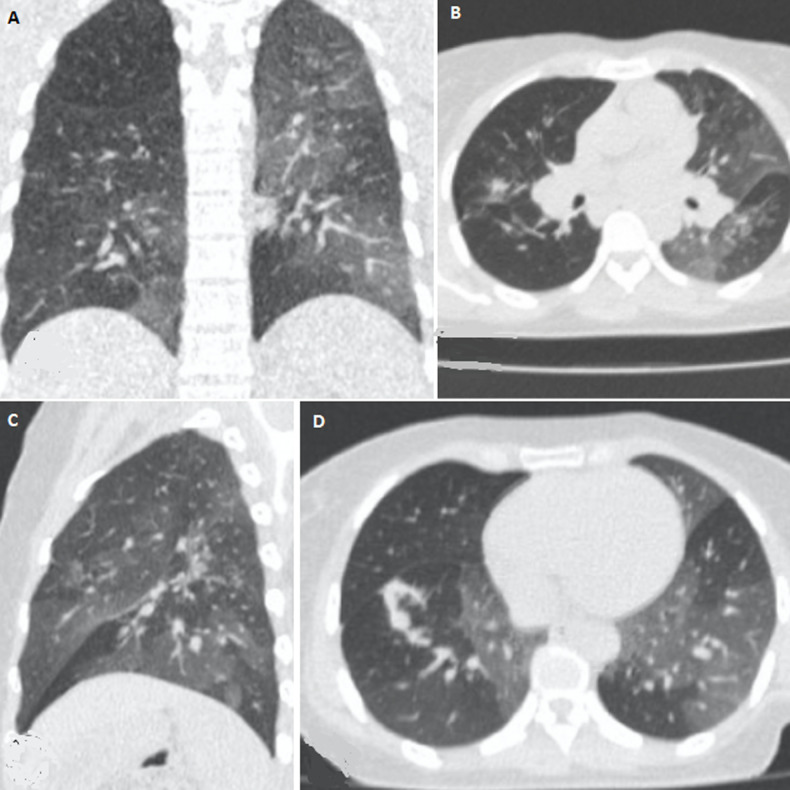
non-contrast Chest CT in lung window: A) coronal reconstruction; B) axial section; C) sagittal reconstruction showing patchy ground-glass, opacities, central and peripheral, bilateral and predominantly on the left; D) axial sections
